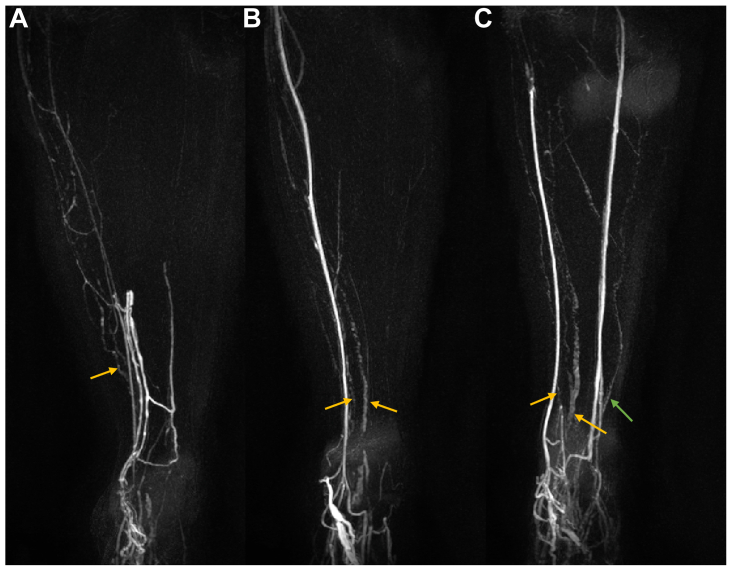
Example images demonstrating a range of vessel numbers and both anteromedial and anterolateral pathways. Coronal subtraction maximum intensity projection (MIP) images for the left leg of three female participants showing one (A), two (B), and three (C) lymphatic vessels observed at the level of the ankle approximately 20 minutes after T1-weighted post-contrast imaging began. C, This leg also demonstrates clear anterolateral lymphatic drainage (*green arrow*). *Orange arrows* indicate anteromedial vessels. All limbs also show clear venous enhancement.
